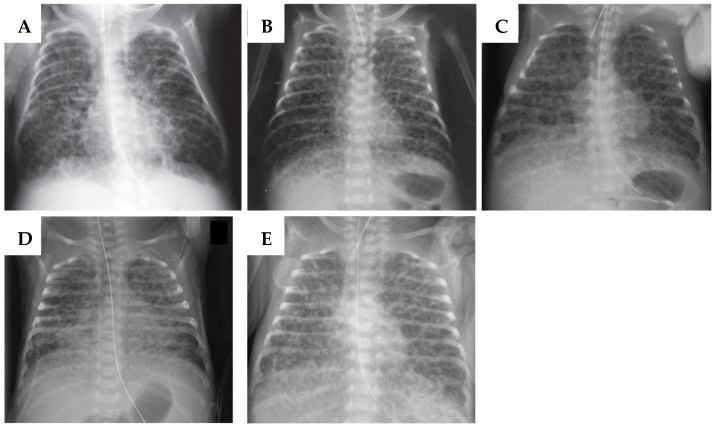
Reference X-rays for the appearance of bubbly/cystic tissue. The X-ray data used in this BPD classification came from the following publications: (A) Arai H. *Acta Paediatr*. 2020 [22]; (B) Namba F. *Pediatr Int*. 2016 [26]; (C) Hirata K. *Arch Dis Child*. 2015 [23]; (D) Muehlbacher T. *Children*. 2021 [25]; (E) Morris S. *Current Paediatrics*. 2003 [24].
